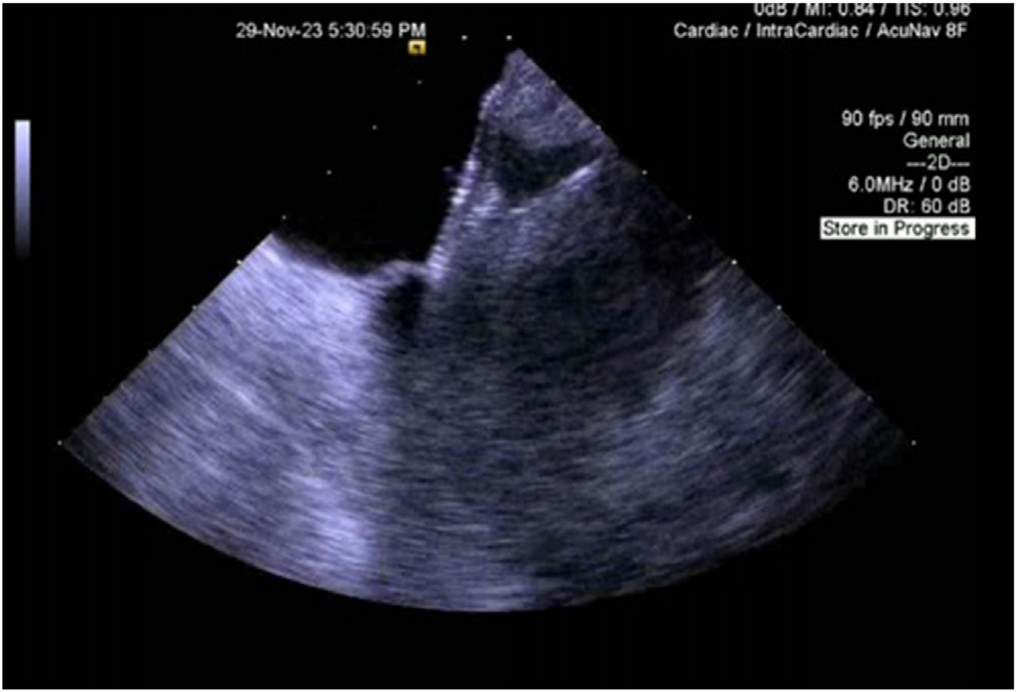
Transesophageal echocardiogram during transcatheter patent foramen ovale closure showing Amplatzer Talisman 35-mm occluder device(Abbott)and successful closure of patent foramen ovale.
